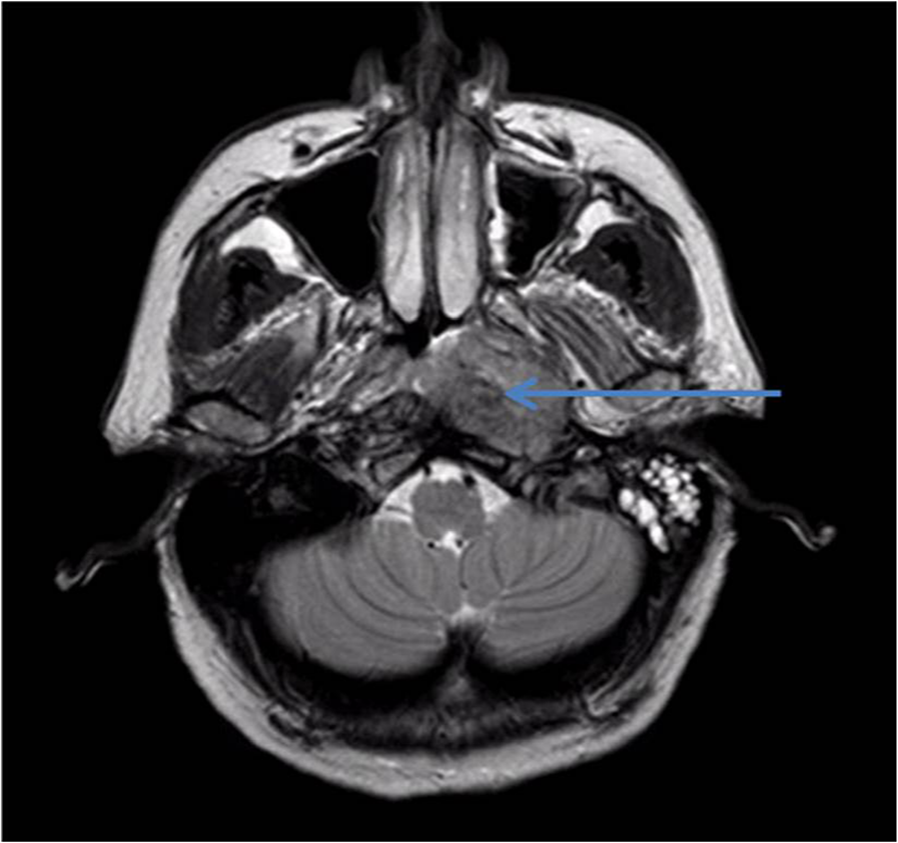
T2-weighted axial magnetic resonance image depicting a left-sided nasopharyngeal mass (arrow) and fluid filling left mastoid air cells.
